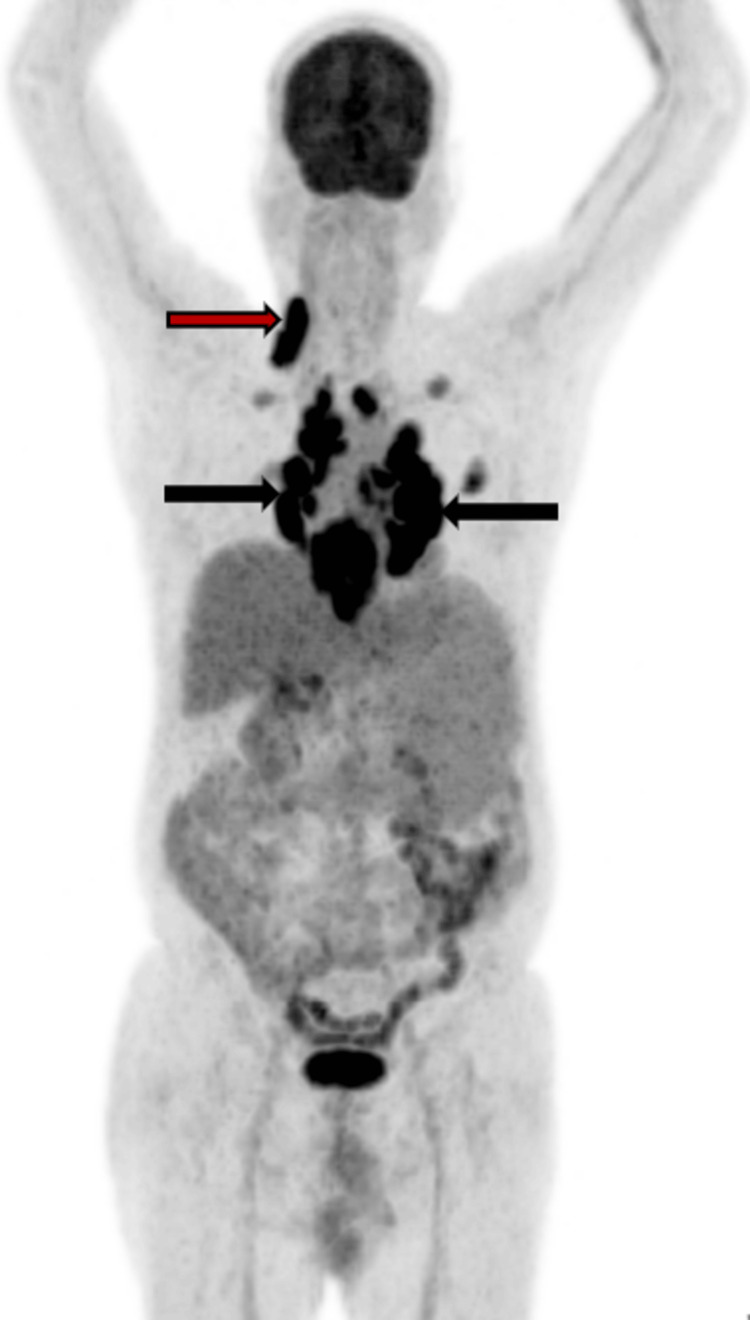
Whole-body ¹⁸F-fluorodeoxyglucose (FDG) positron emission tomography (PET) maximum intensity projection image demonstrating intense FDG uptake in upper right cervical lymph nodes and bilateral hilar lymph nodes as shown by the red and black arrows, respectively
